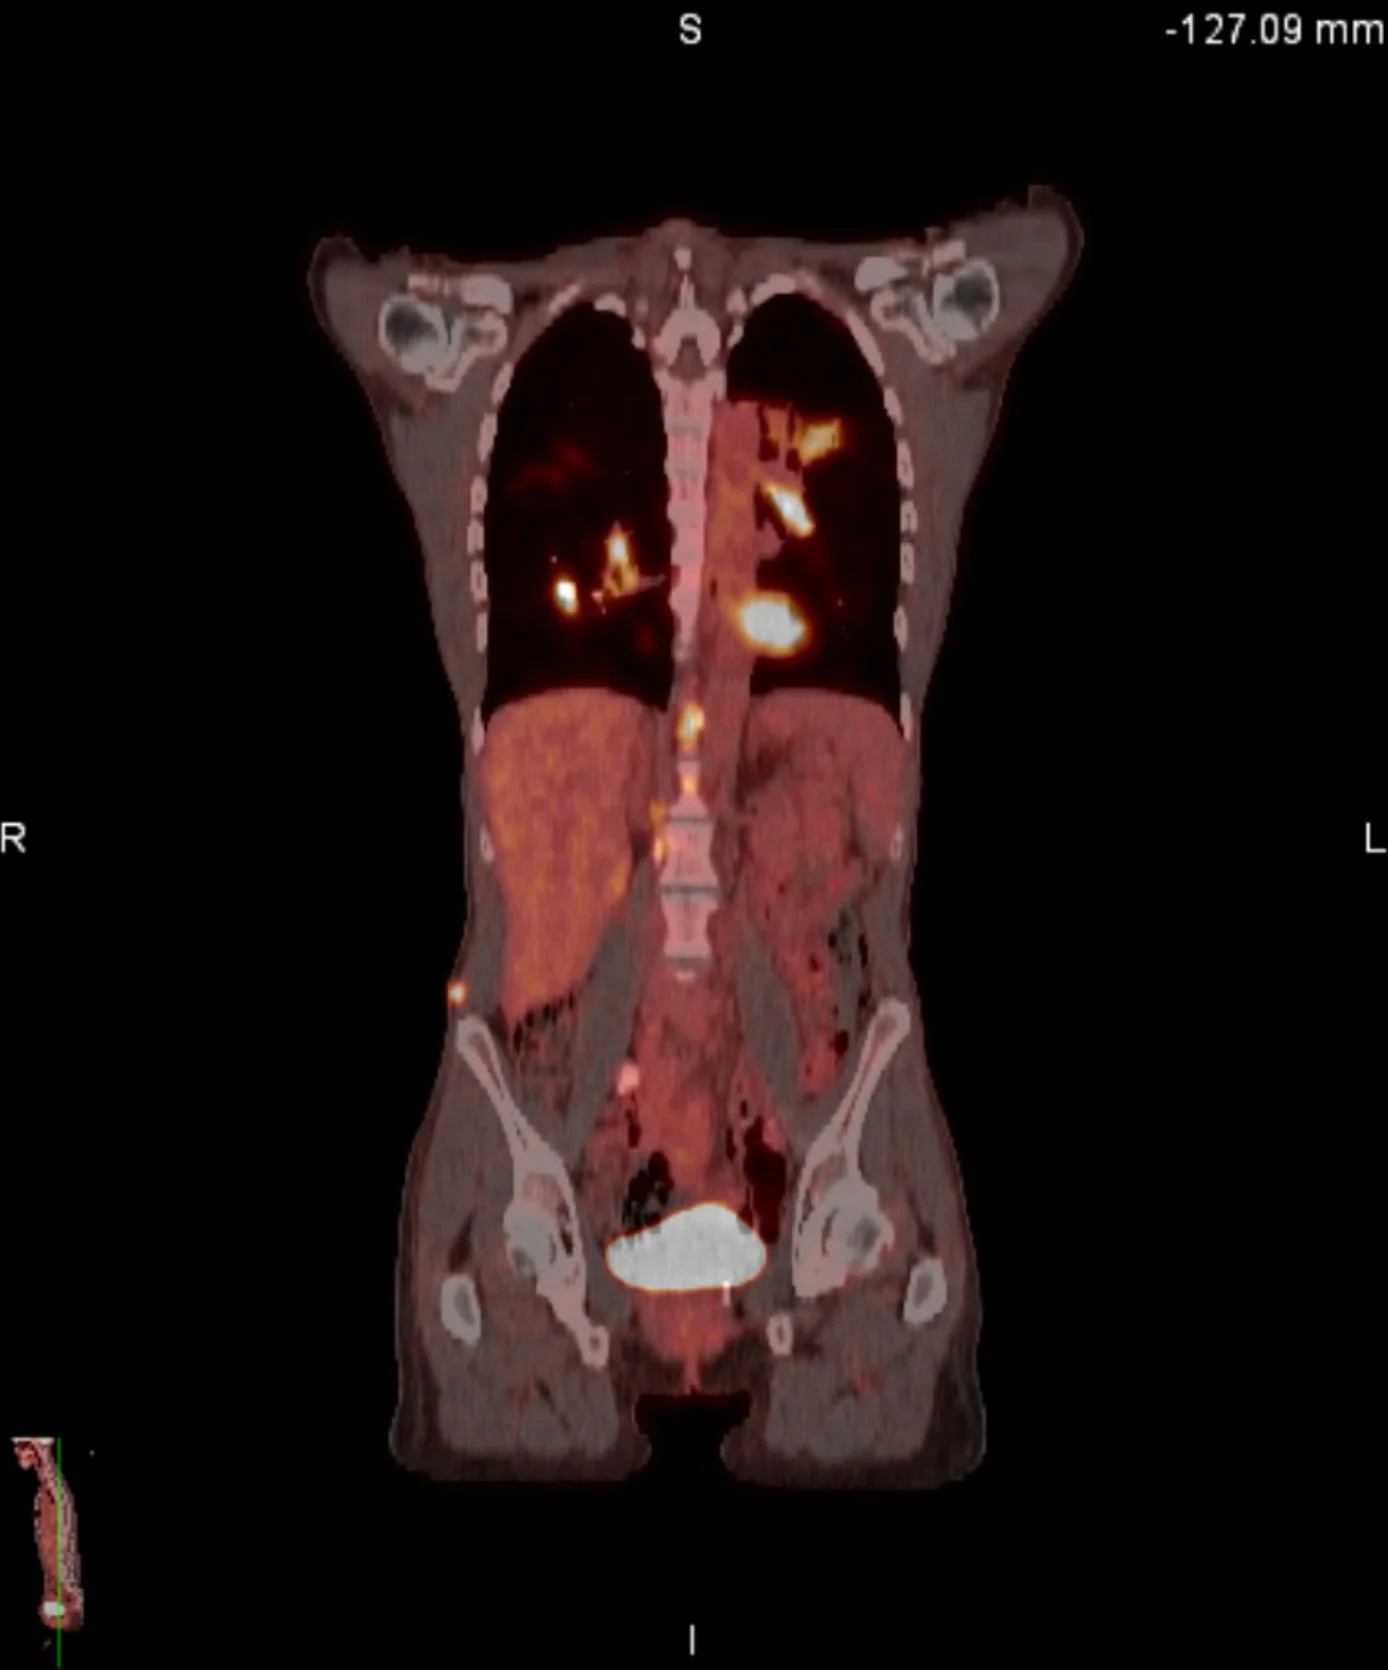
Whole body PET-CT showing widespread metastases to lung, lymph nodes, and skin.
